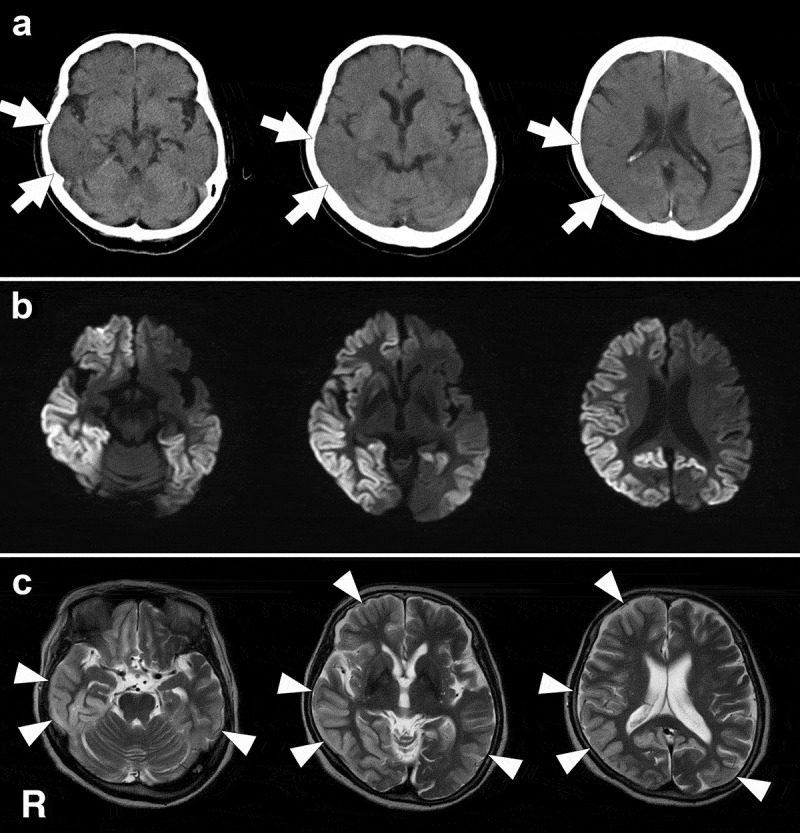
Head computed tomography (CT) and magnetic resonance imaging (MRI) findings. These images were obtained 8 months after the disease onset. Clinically, facial mimicry and pathological laughing were remarkable at this stage. a: CT images show edematous and swelling findings in the right cerebral hemisphere, particularly in the temporal and parietal lobes (arrows). In the right temporal and parietal cortices, the sulcus shows narrowing and the corticomedullary junction is generally indistinct. b: Diffusion weighted MRI shows widespread gyriform hyperintensity in the right cerebral hemisphere and left parietal and occipital lobes, except in the medial occipital regions. c: T2-weighted images show hyperintense regions with swelling (arrowheads), and the regions correspond to those that were hyperintense on diffusion weighted MRI. R, right side.
